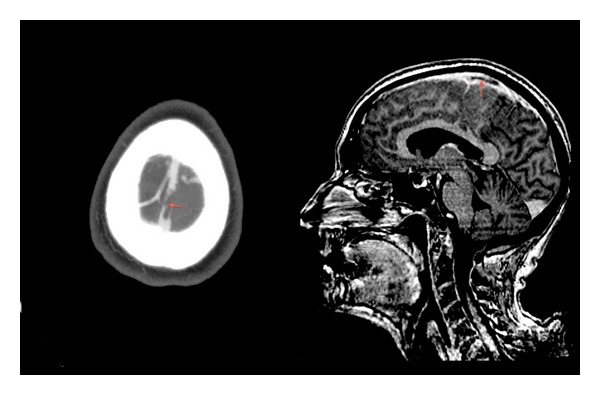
Brain computed tomography showing superior sagittal sinus thrombosis and implication of a cortical vein (arrows).
